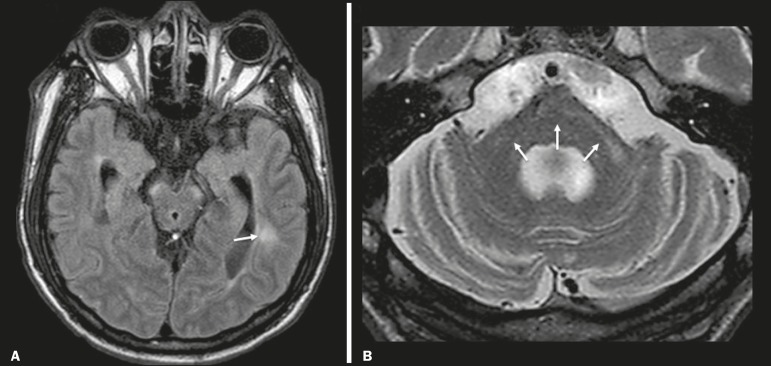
CARASIL. A: Axial FLAIR sequence showing a hyperintense signal in the periventricular portion of the left temporal lobe. B: Axial T2-weighted sequence showing an arc-shaped hyperintense signal in the pons (“split pons sign”). In a T2*-weighted sequence, there were areas with hemosiderin deposits (not shown).
